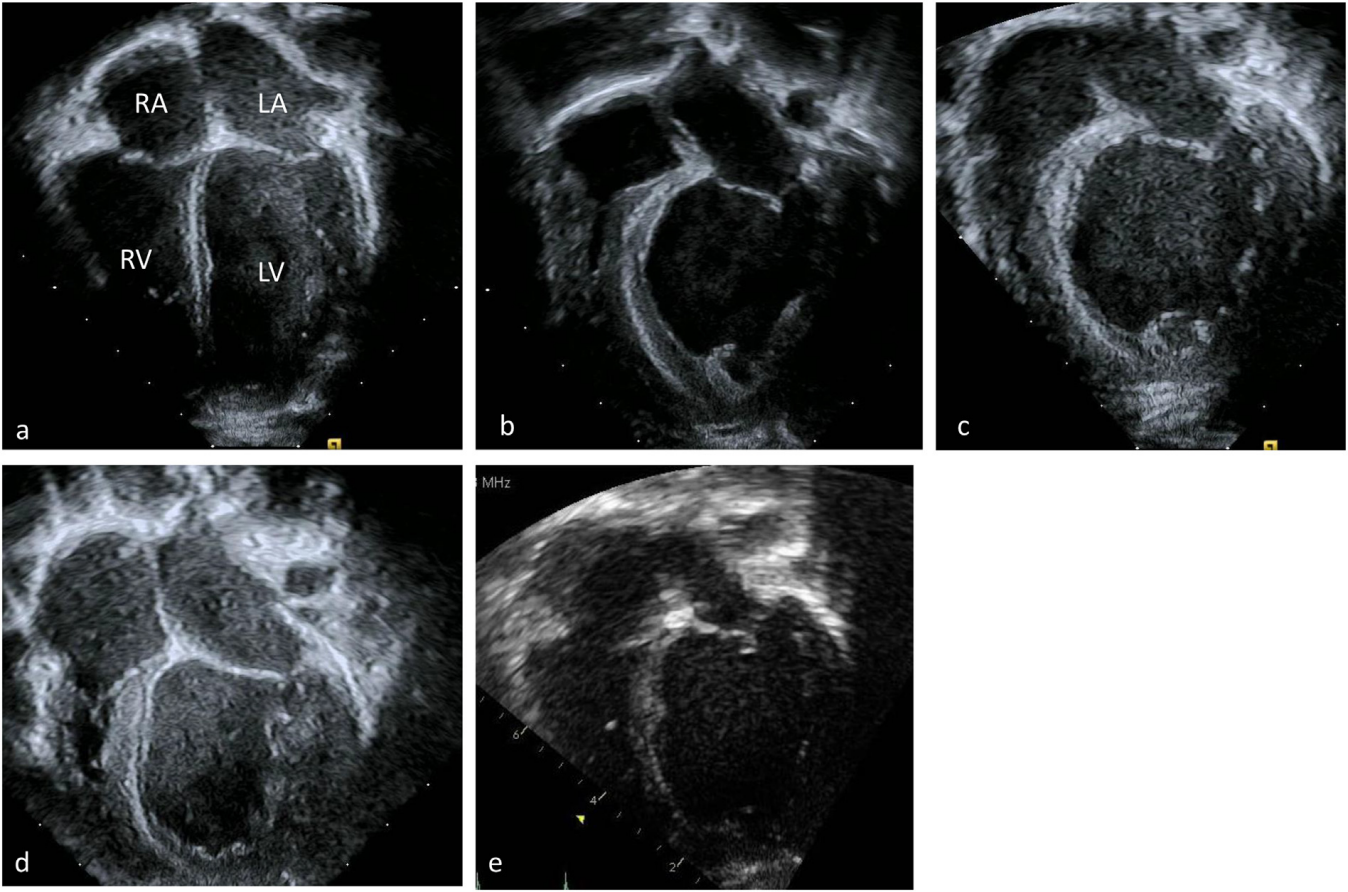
Four chamber apical echocardiographic view of a (a) normal heart with a similar view of this patient's hearts at (b) time of diagnosis (c) two months after initiation of ERT (d) four months after initiation and (e) a year after initiation. These images emphasize the dilation of this patient's left ventricle. RA right atrium, LA left atrium, RV right ventricle, LV left ventricle.
